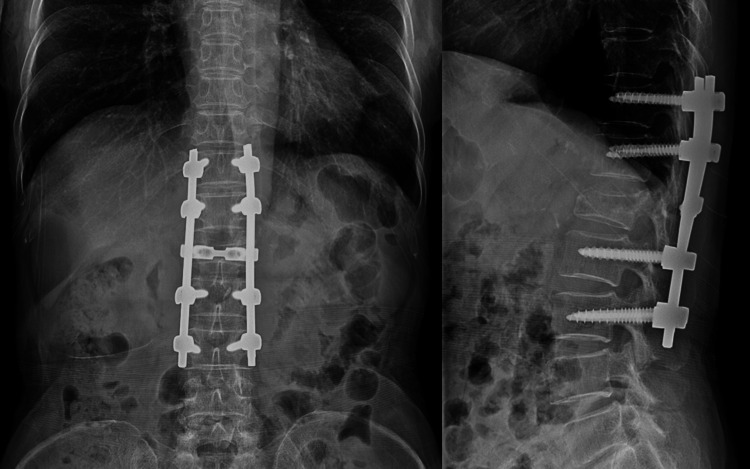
Plain radiographs 18 months after the surgery.
